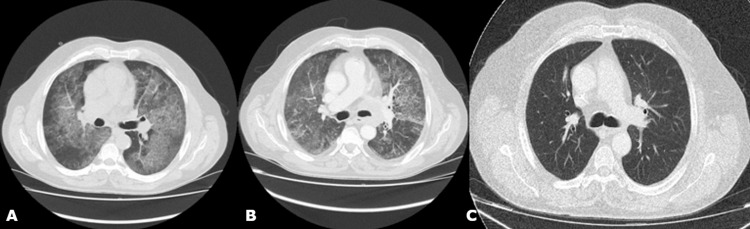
A. Chest computed tomography on admission. B. Radiological improvement three weeks after whole lung lavage and supplementation therapy with inhaled sargramostim. C. Further radiological improvement at six-month follow-up.
